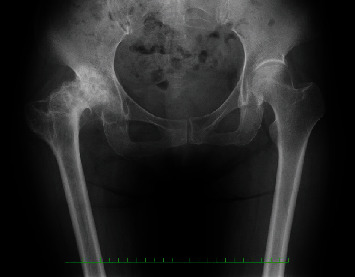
Radiographs of Case 1 before total hip arthroplasty.
